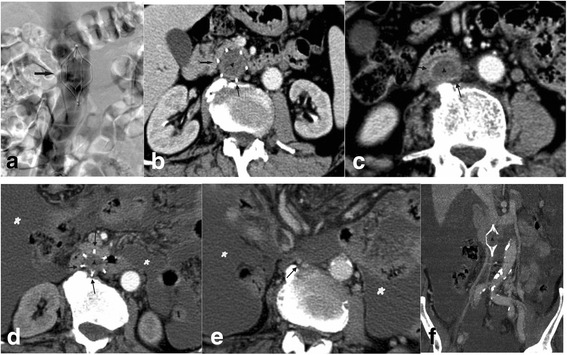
Patient with a typical case of chronic liver cirrhosis who developed a left-sided iliofemoral thrombosis. a An OptEase filter (black arrow) was placed in the inferior vena cava (IVC). b, c Follow-up computed tomography (CT) of the IVC performed 14 days after filter placement revealed a “filling defect” in and below the filter (black arrowhead), indicating extensive intra-filter and IVC thrombosis. The thickened IVC wall (black arrow) appeared as a ring around the thrombus (c). d–f The last CT imaging of the IVC was performed 14 months after filter placement. d An intra-filter thrombus (black arrowhead). The protruded struts of the filter (black arrow) indicate grade 2 protrusion. Large amount of ascites (black asterisk) was also present. e, f The IVC below the filter shows as an “atrophic” strip (black arrow)
